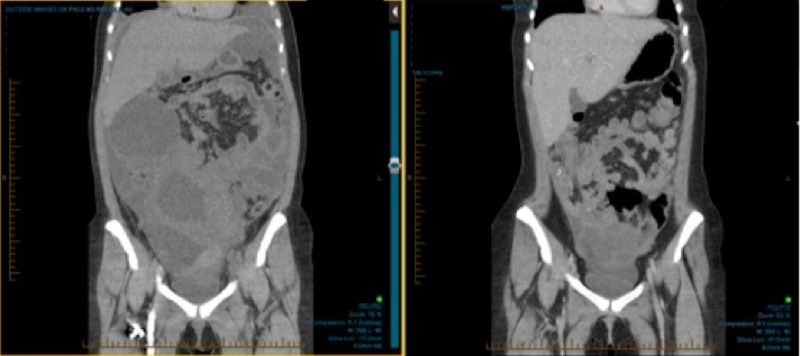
Coronal CT scan prior and after chemotherapy. Comparison between staging CT scan and after the 6th cycle of chemotherapy, revealing significant regression with two residual lesions: one in the right flank measuring 4.5 cm in greatest dimension and one in the pelvis measuring 10.7 cm in greatest dimension.
